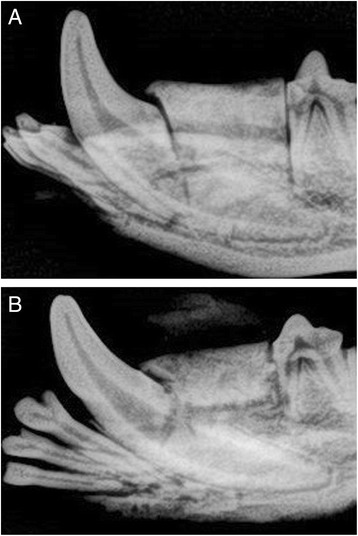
a Peri-apical X-ray of zirconia scaffold immediately placed in bone defect. Margins between scaffold and bone are clearly demarcated. b Peri-apical X-ray of zirconia scaffold after completion of healing time. Margins between bone defect and scaffold are less demarcated due to new bone growth
